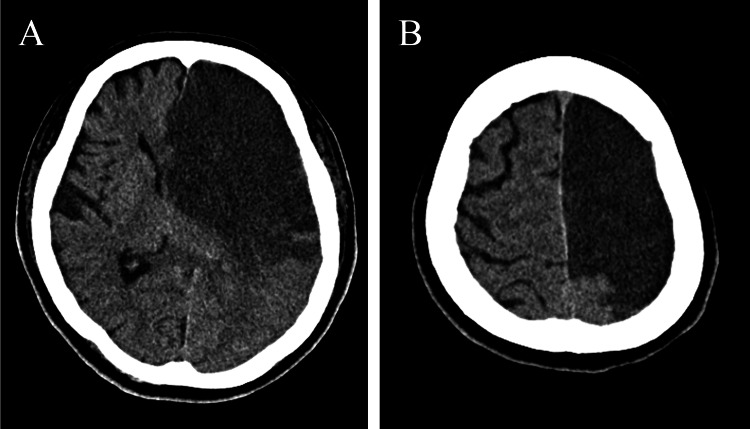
(A and B) Brain computed tomography on deterioration in condition after treatment. Expanding acute cerebral infarction lesion in the left internal carotid artery (A and B).
